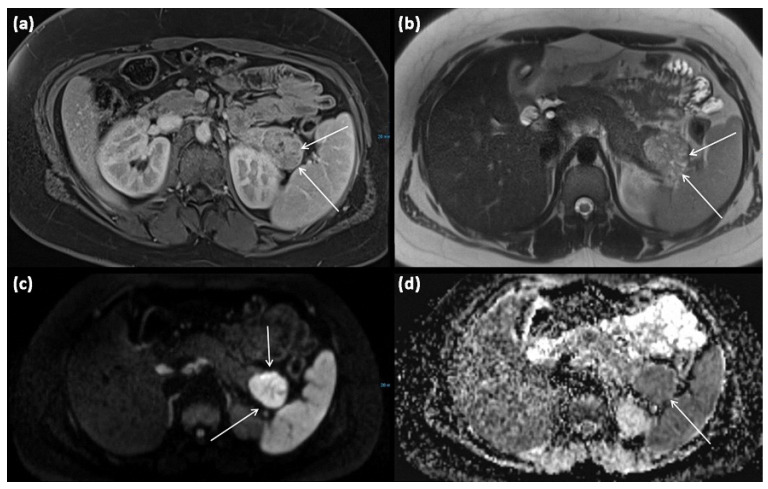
MRI of a 15-year-old female patient with axial T1w after gadolinium-based contrast administration (a) T2w (b) as well as DWI (diffusion-weighted imaging) weighting ((c), b = 1000) and ADC map (apparent diffusion coefficient) (d). MRI shows a solid tumor of the pancreatic tail. In T2w, almost exclusively, intermediate to hypointense signals can be seen, showing high cellularity in the absence of cystic parts. There is a strongly hyperintense signal in DWI with a signal decrease in ADC. The tumor is marked with arrows in all sequences.
